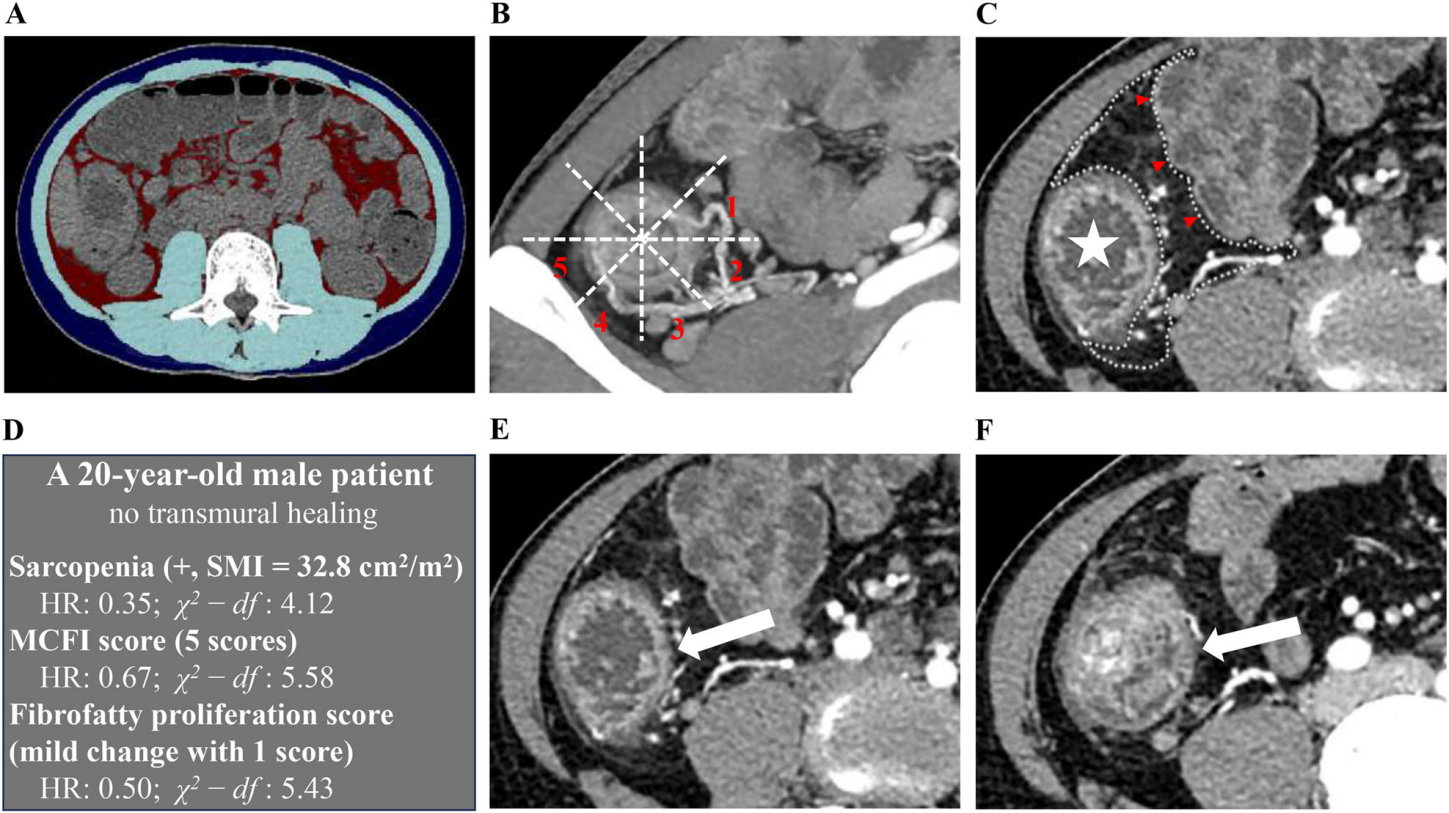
A patient who experienced a failure to achieve TH (A–F). A Axial non-contrast CT image at the L3 level shows the segmentation of skeletal muscle (light blue), visceral adipose tissue (red), and subcutaneous adipose tissue (dark blue). The patient was diagnosed with sarcopenia, with an SMI of 32.8 cm^2^/m^2^. B, C The MCFI and fibrofatty proliferation score of the ascending colon (indicated by the star) are scored as 5 and 1, respectively. E Axial post-contrast CT image shows the pretreatment inflammation of the ascending colon (arrow). F After 74 weeks of UST treatment, this patient experienced a failure to achieve TH, with BWT > 3 mm, mural hyperenhancement, and mesenteric edema. TH, transmural healing; L3, third lumbar vertebra; MCFI, mesenteric creeping fat index; SMI, skeletal muscle index; UST, ustekinumab; BWT, bowel wall thickness
